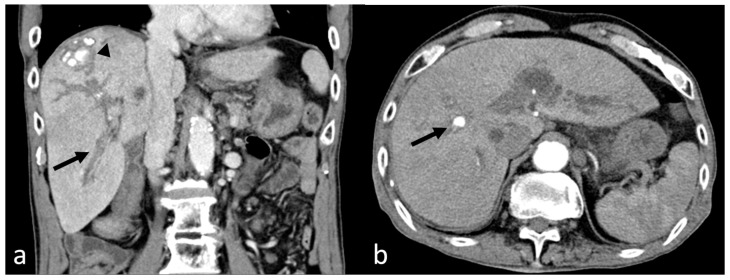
(a) The coronal computed tomography (CT) image reveals hemobilia in the right intrahepatic duct (arrow) and hepatocellular carcinoma (arrowhead) in liver segment 8, previously treated with transarterial chemoembolization (b) The axial CT image shows a pseudoaneurysm (arrow) in the hepatic artery of segment 8.
